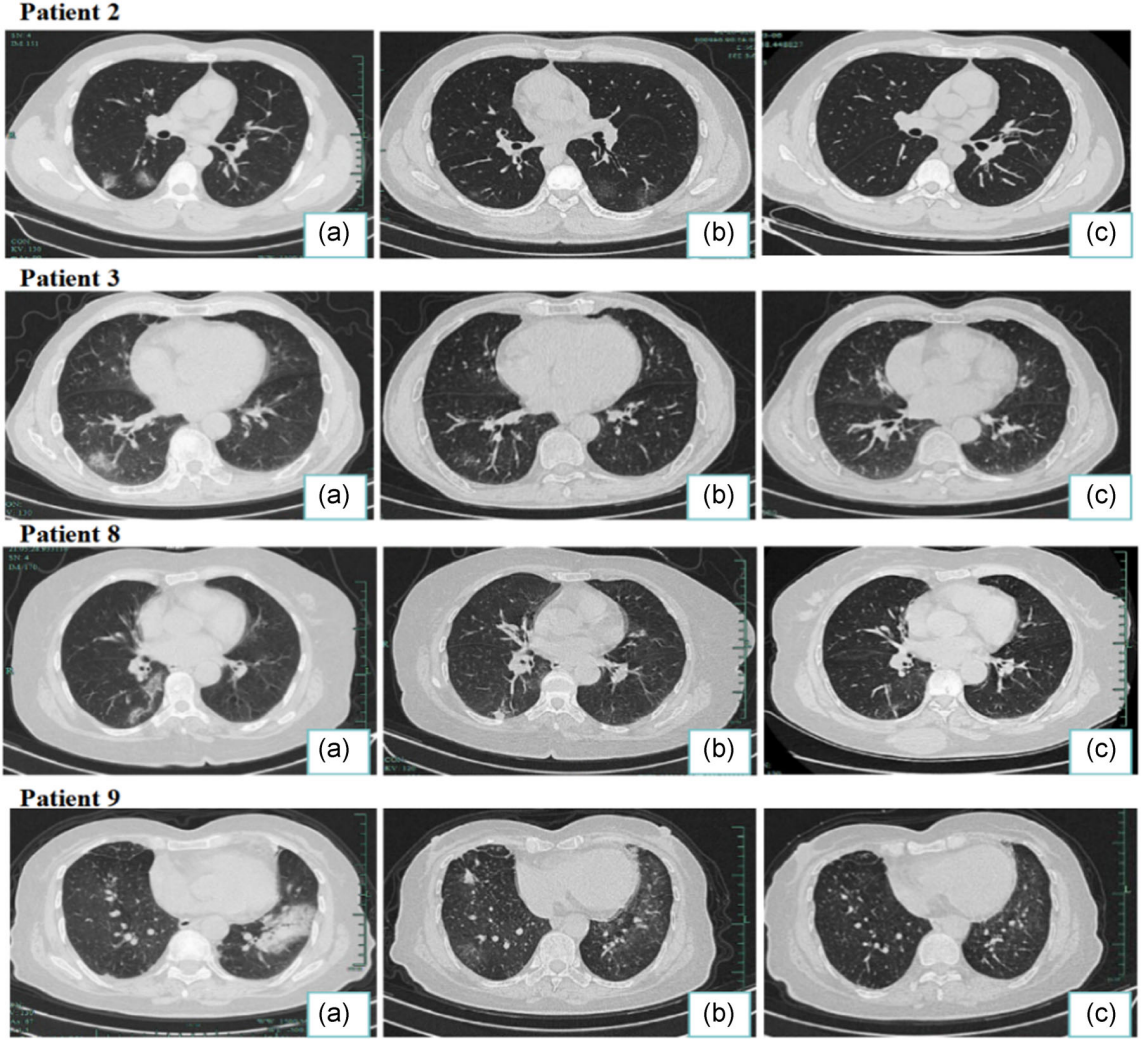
Transverse chest CT images from COVID‐19 patients with recurence of SARS‐CoV‐2. A, During hospitalization, CT scans typical signs of COVID‐19: density‐increased patchy consolidation and ground‐glass shadow. B, CT scans while patient had met the current discharge standards:strip high‐density shadows of the lung with largely absorbed. C, Lung CT scans at the time of nucleic acid recurence: similar to (B). CT, computed tomography
